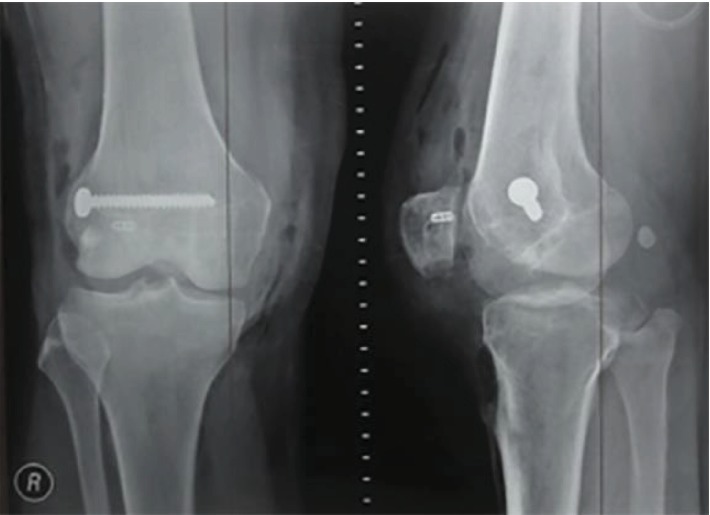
Radiographic examination to confirm the position of the endobutton, cancellous screw, and washer postoperatively.
